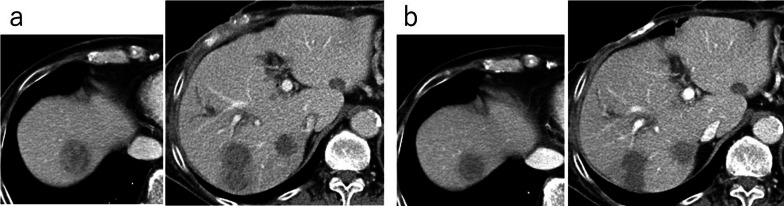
Contrast-enhanced CT showing the therapeutic effect of PR after six courses of GC. a Before chemotherapy. b After six courses of GC
